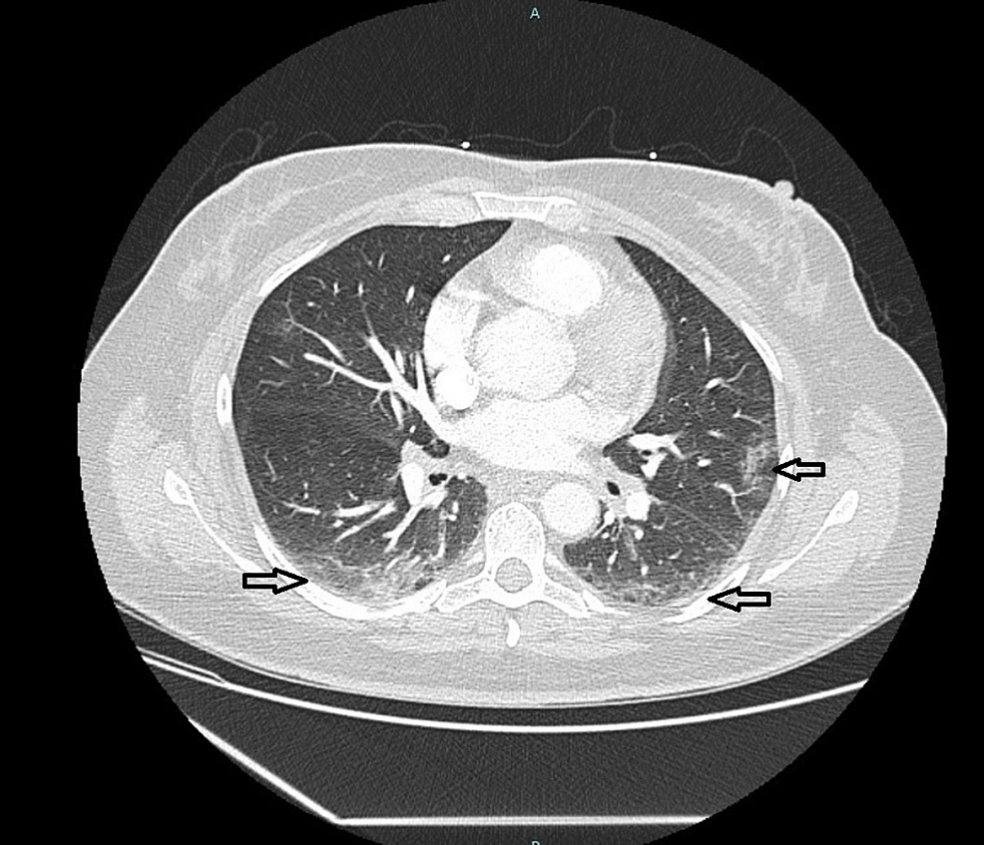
CT scan of the chest with contrast showing bilateral ground-glass opacities consistent with COVID-19 infection. CT, computed tomography; COVID-19, coronavirus disease 2019
